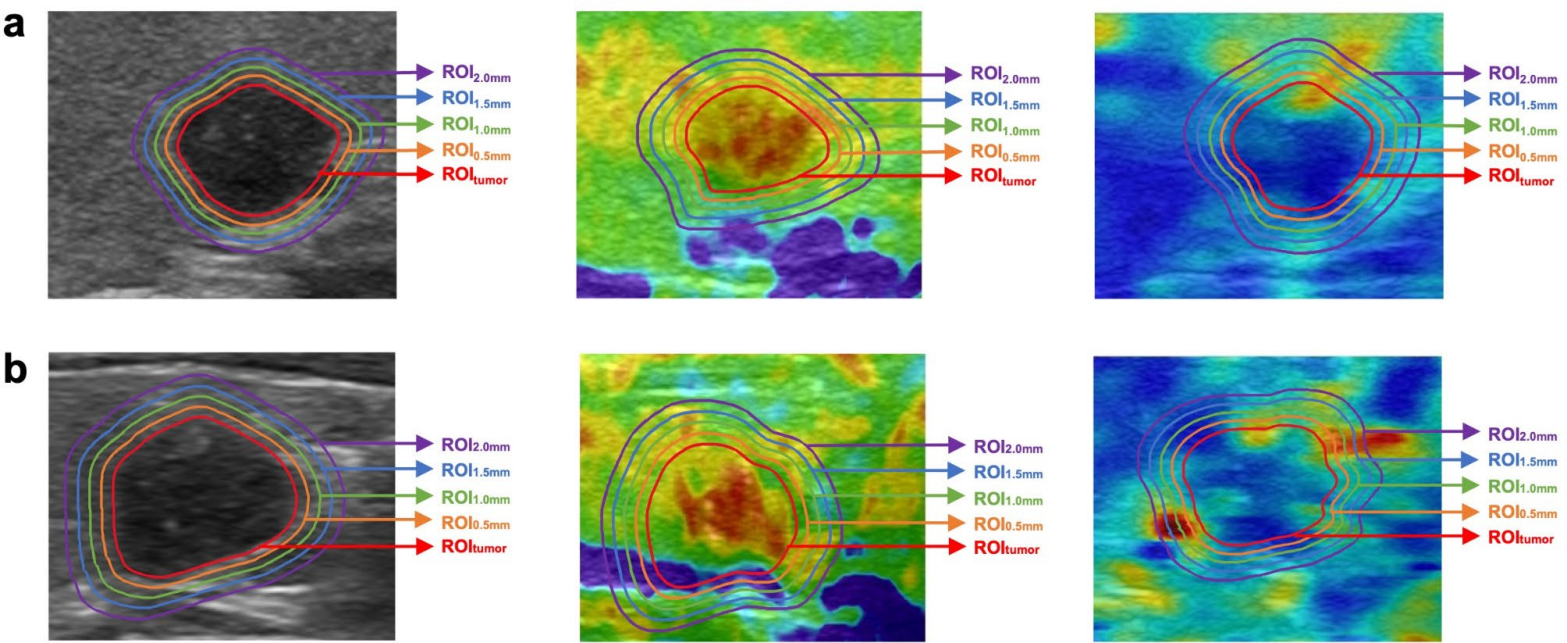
Tumor segmentation and peritumoral expansion of two representative examples on BMUS, SE and SWE images. The ROI_tumor_ represents the intratumoral region. The ROI_0.5 mm_, ROI_1.0 mm_, ROI_1.5 mm_ and ROI_2.0 mm_ represent the combined region including both the intratumoral and peritumoral areas with 0.5–2.0 mm widths. (a) A 39-year-old female without cervical LNM; (b) A 50-year-old female with cervical LNM. BMUS: B-mode ultrasound; LNM: lymph node metastasis; ROI: region of interest; SE: strain elastography; SWE: shear wave elastography
